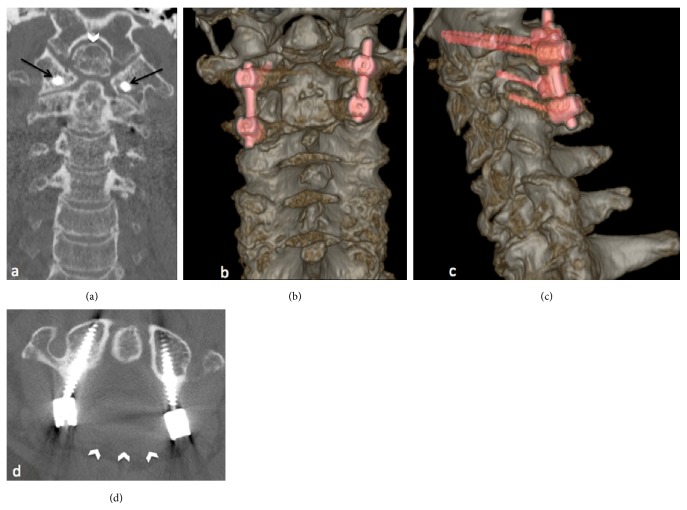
Unenhanced CT after posterior C1–C2 fixation. We see in coronal plane with bone window the transarticular C1-C2 screws (a, black arrows) and on volume rendering in coronal and sagittal reconstructions (b,c, the screws are highlighting). Spinal canal decompression by laminectomy is also made, seen in axial plane with bone window (d, white arrowheads) showing the absence of posterior arch of C1 (in comparison to image c of Figure 1). The intracanal ROP is not removed but regressed in size compared to preoperative CT.
